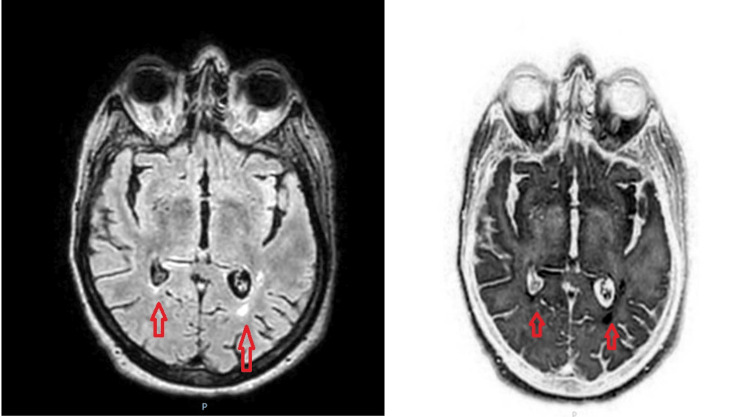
Repeat magnetic resonance imaging (MRI) of the brain and T2 sagittal and coronal sequences indicated persistent pyogenic ventriculitis.
